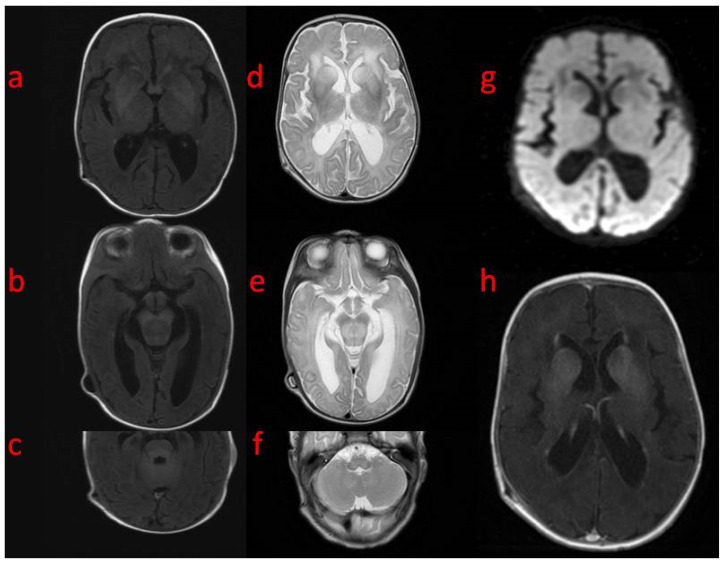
Brain MRI (a–c) (5th week of life): axial T1 on the level of (a) basal ganglia, (b) midbrain, (c) pons with superior cerebellar peduncles with an abnormal signal in the frontal white matter, basal ganglia without signal changes in the pons and cerebellum (d–f): axial T2—(d) significantly increased frontal white matter and basal ganglia signal, enlarged caudate nuclei, (e) increased signal and volume in the midbrain, (f) increased signal in the oblongate medulla; (g) axial DWI—generalized even signal without differentiation except for increased diffusivity in the frontal white matter; (h) axial T1 post-contrast: enhancement of basal ganglia.
